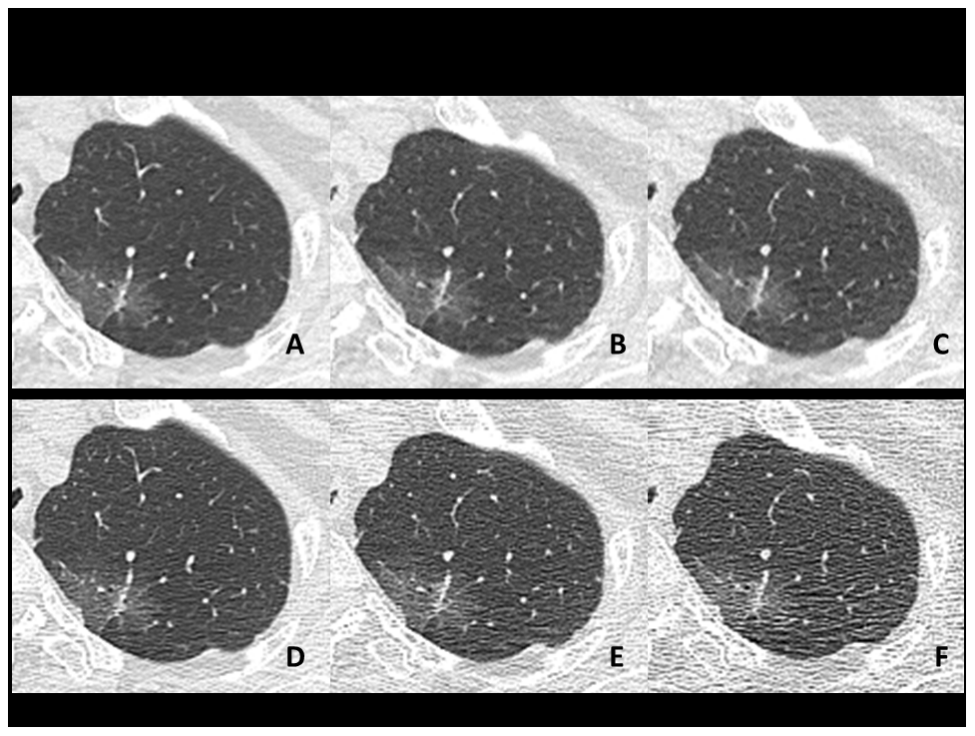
Axial plain chest CT images showing a ground-glass opacity (GGO) nodule in the left apex (74-year-old female weighing 49 kg). Images are arranged as in Figures 1 and 2. Nearly homogeneous density of the nodule was accurately depicted on images with AIDR3D at any of three tube currents (A–C). However, on images without AIDR3D (D–F), artificial density heterogeneity due to image noise increased as the tube current decreased from 240 (D) to 60 mA (F).
